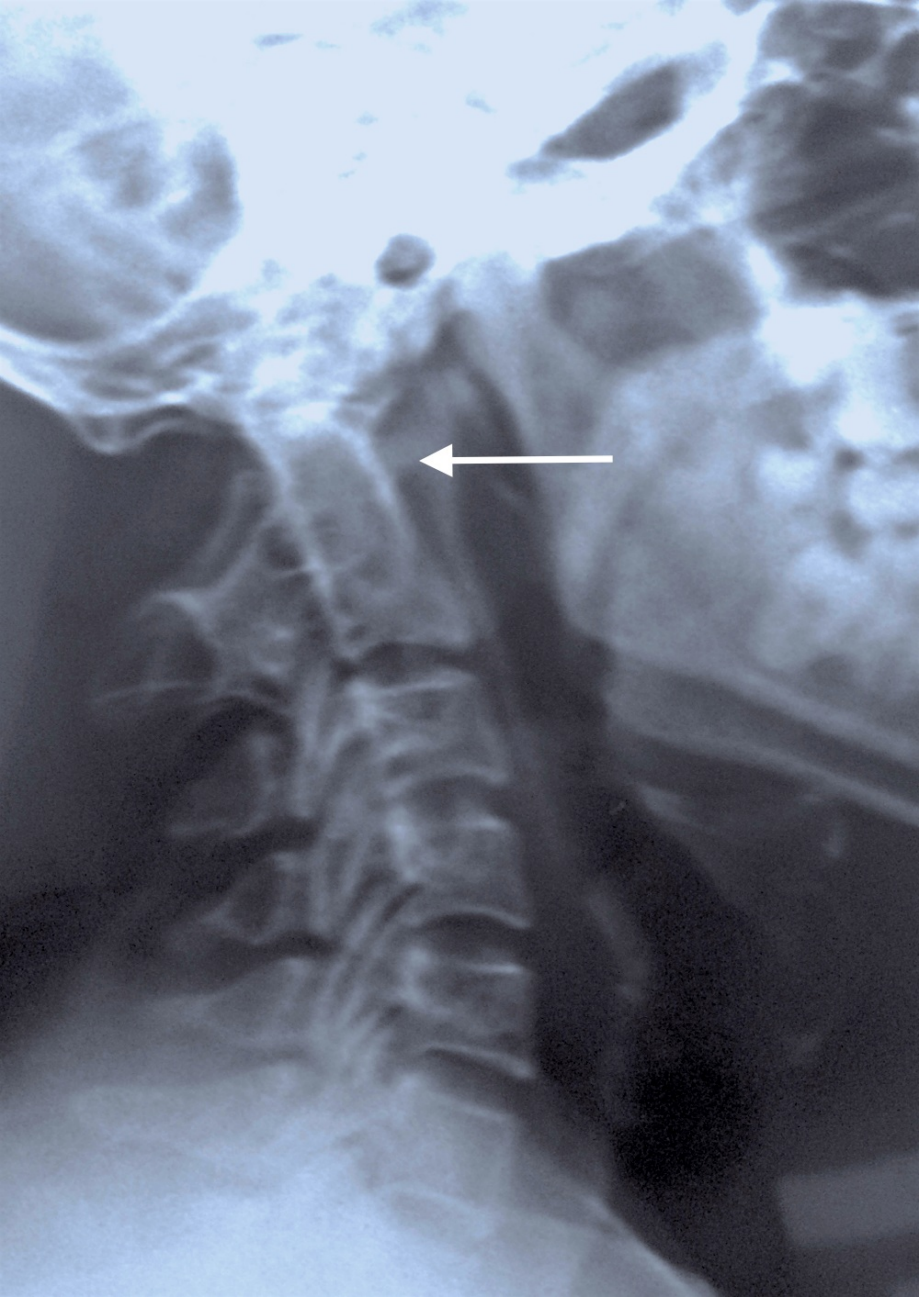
Lateral radiograph of the cervical spine falsely interpreted as "congenital absence of atlas" (arrow)
